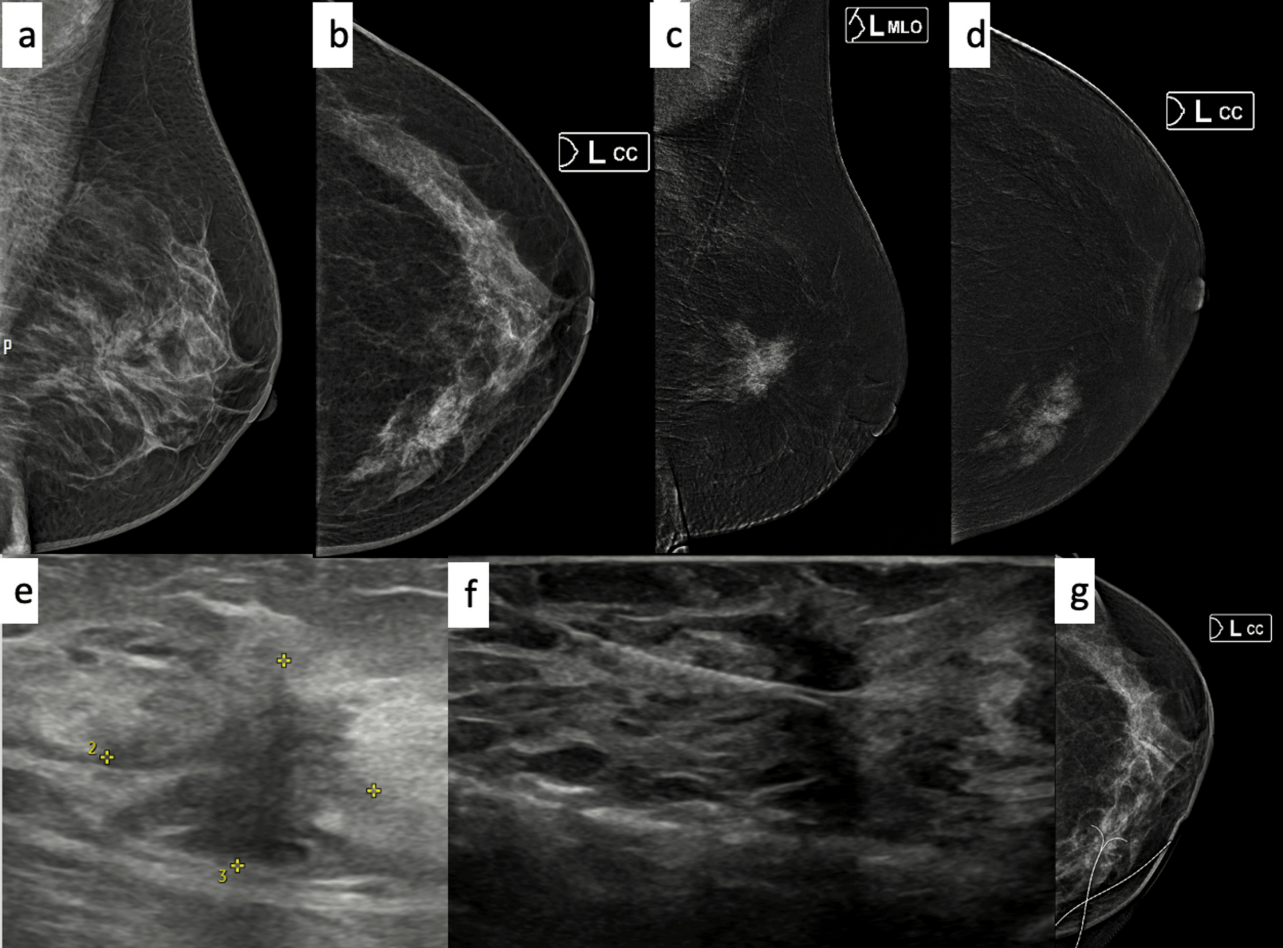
A case of a 32-year-old female a,b: X-ray mammogram MLO and CC views of the left breast show an architectural distortion (AD) with an underlying irregular mass in the inner central quadrant; c,d: CEDM recombined images show heterogeneous enhancement in the region of AD and mass; e: ultrasound shows an irregular taller-than-wide spiculated mass with surrounding AD; f,g: ultrasound-guided wire localization with a check X-ray mammogram. CC: craniocaudal; MLO: mediolateral oblique; CEDM: contrast-enhanced digital mammography
